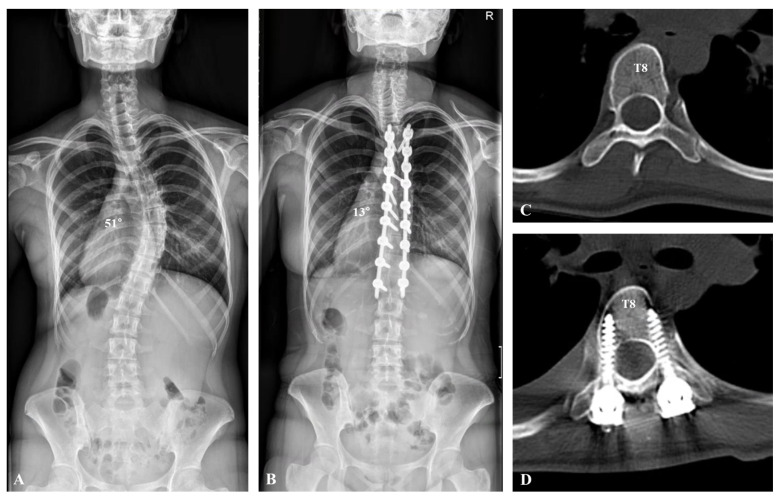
A 14-year-old female patient visited the hospital for progressive spinal deformity. (A) A whole-spine anteroposterior image showed 51° of scoliosis deformity with T8 apical vertebra. (B) Deformity correction from T5 to L1 was performed with polyaxial pedicle screws and rod derotation, resulting in a correction from 51° to 13°. (C,D) Axial view of computed tomography presented the apical vertebra (T8) before and after deformity correction.
